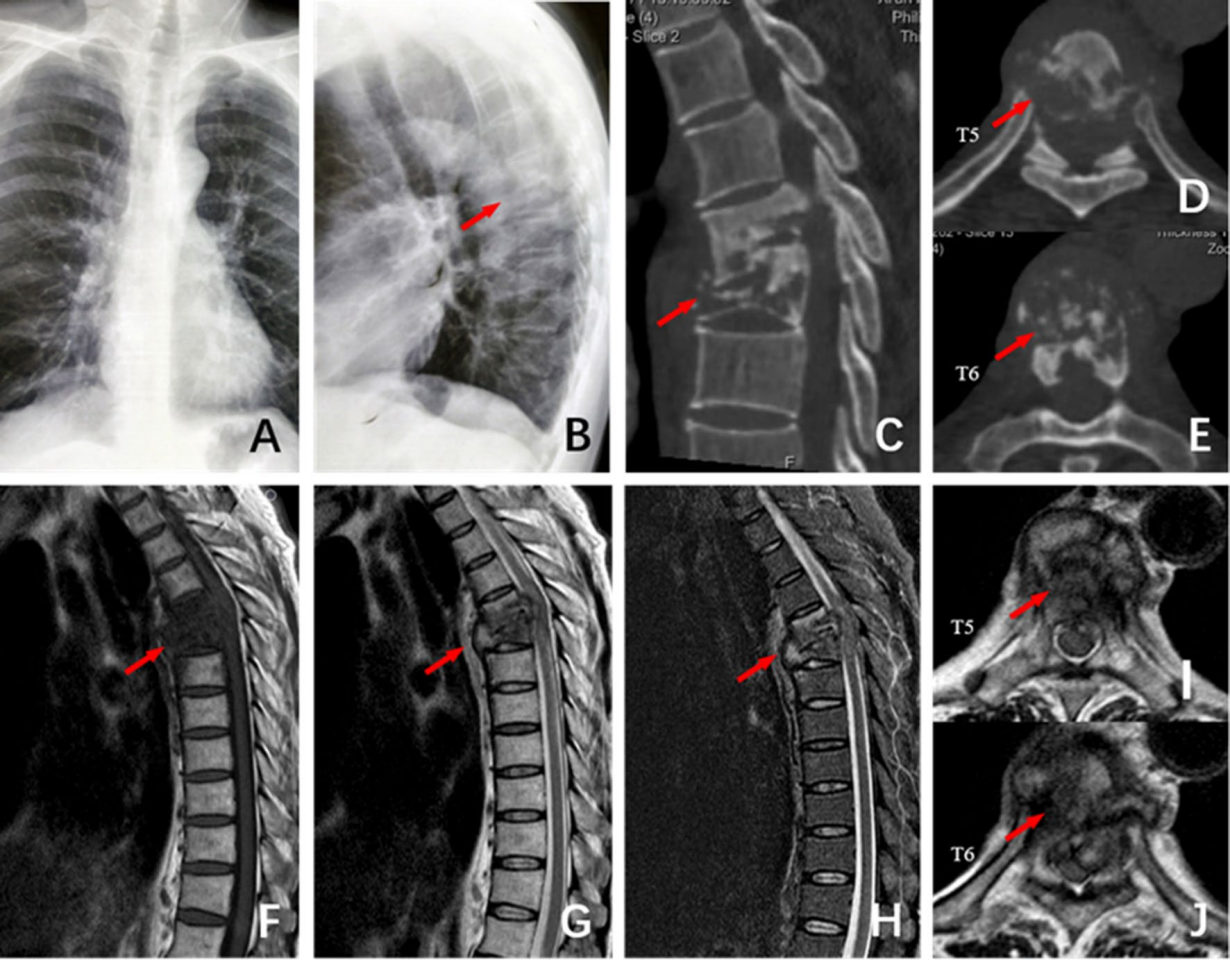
The patient, male, 51 years old, T5 ~ T6 spinal tuberculosis secondary to kyphosis deformity. (A) and (B) The frontal and lateral X-ray films before surgery showed vertebral destruction of T5 ~ T6 and Cobb Angle of 40°. (C–E) Preoperative CT showed severe damage to the anterior column of the T5 ~ T6 vertebral body and normal posterior column. (F–J): Preoperative MRI showed vertebral destruction of T5 ~ T6 and obvious compression of the spinal cord.
